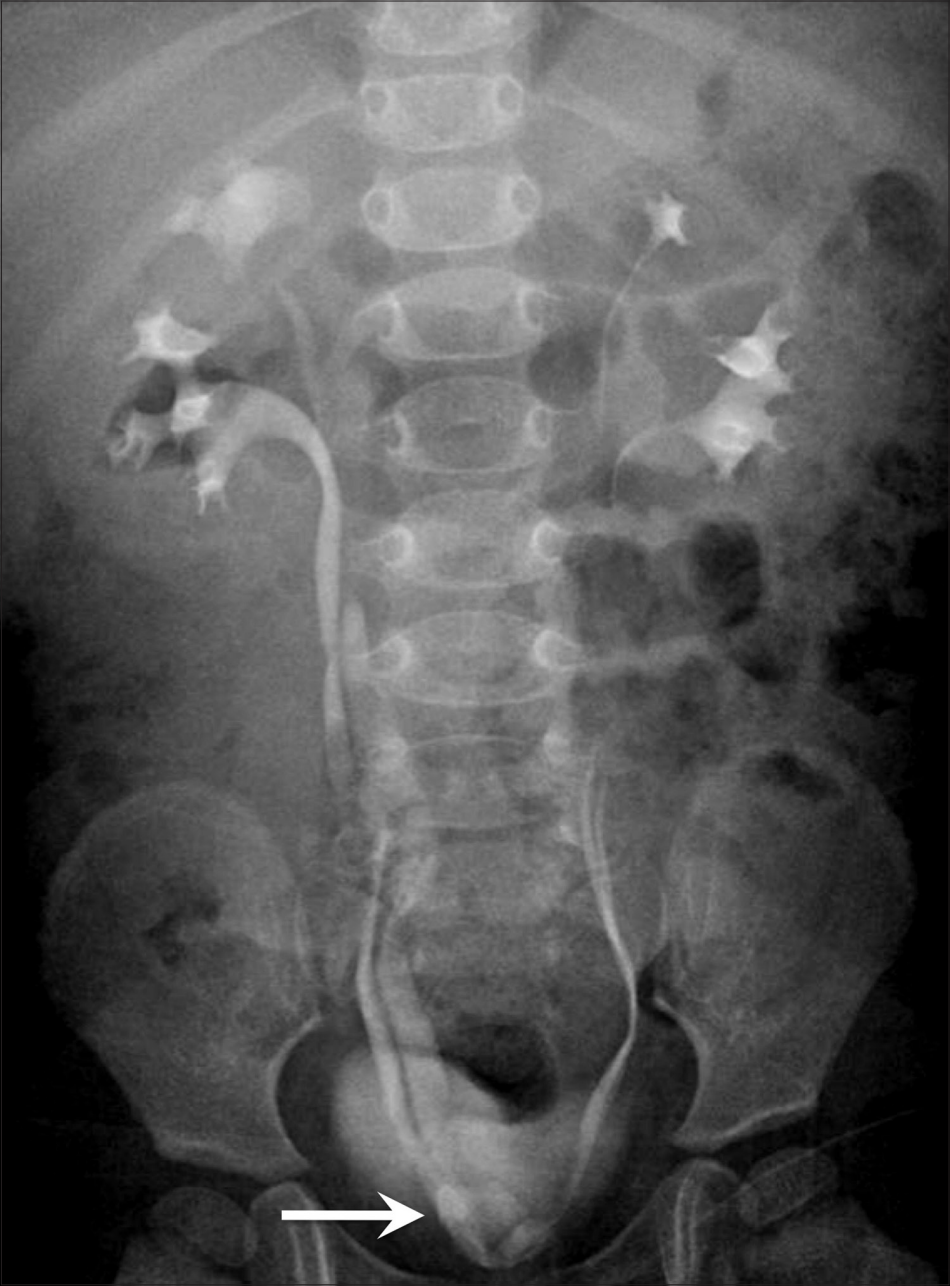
Ureteral duplication: Frontal radiograph from an intravenous urogram study shows bilateral complete ureteral duplication. The opening of the right upper moiety ureter is medial to the lower moiety ureter; there is ureterocele formation
